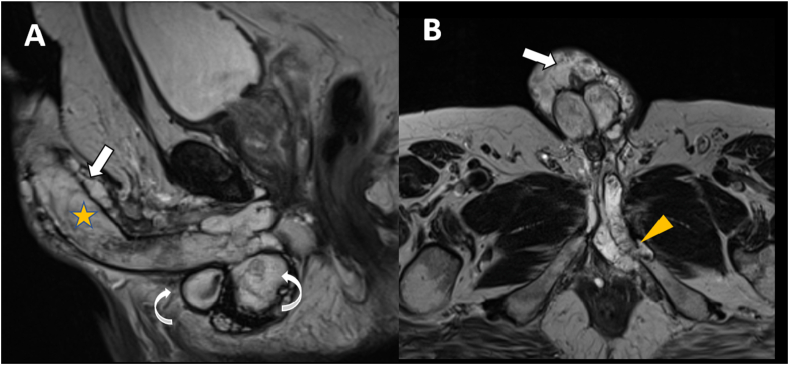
Magnetic Resonance Imaging of the pelvis. A, Sagittal T2 weighted and B, axial T2 weighted MR images showing diffuse infiltrative T2 bright signal intensity lesion along the whole penile shaft (yellow star) extending into superficial extra-albugineal tissue (white bold arrows) and involving the left inferior pubic ramus (yellow arrowhead). In addition, multiple lobulated T2 bright signal intensity lesions inferior to the penile root (curved white arrows). (For interpretation of the references to colour in this figure legend, the reader is referred to the Web version of this article.)
